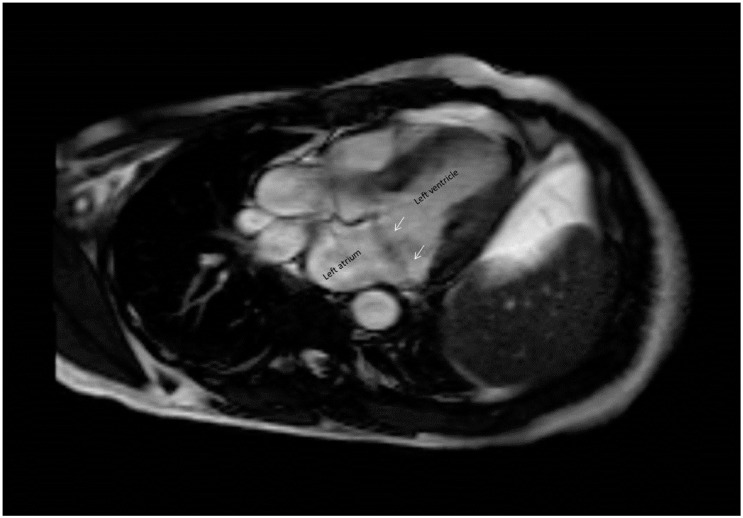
A steady state free precession image on parasternal long axis view of the mitral valve. Note the atrial displacement of both leaflets (arrows).
